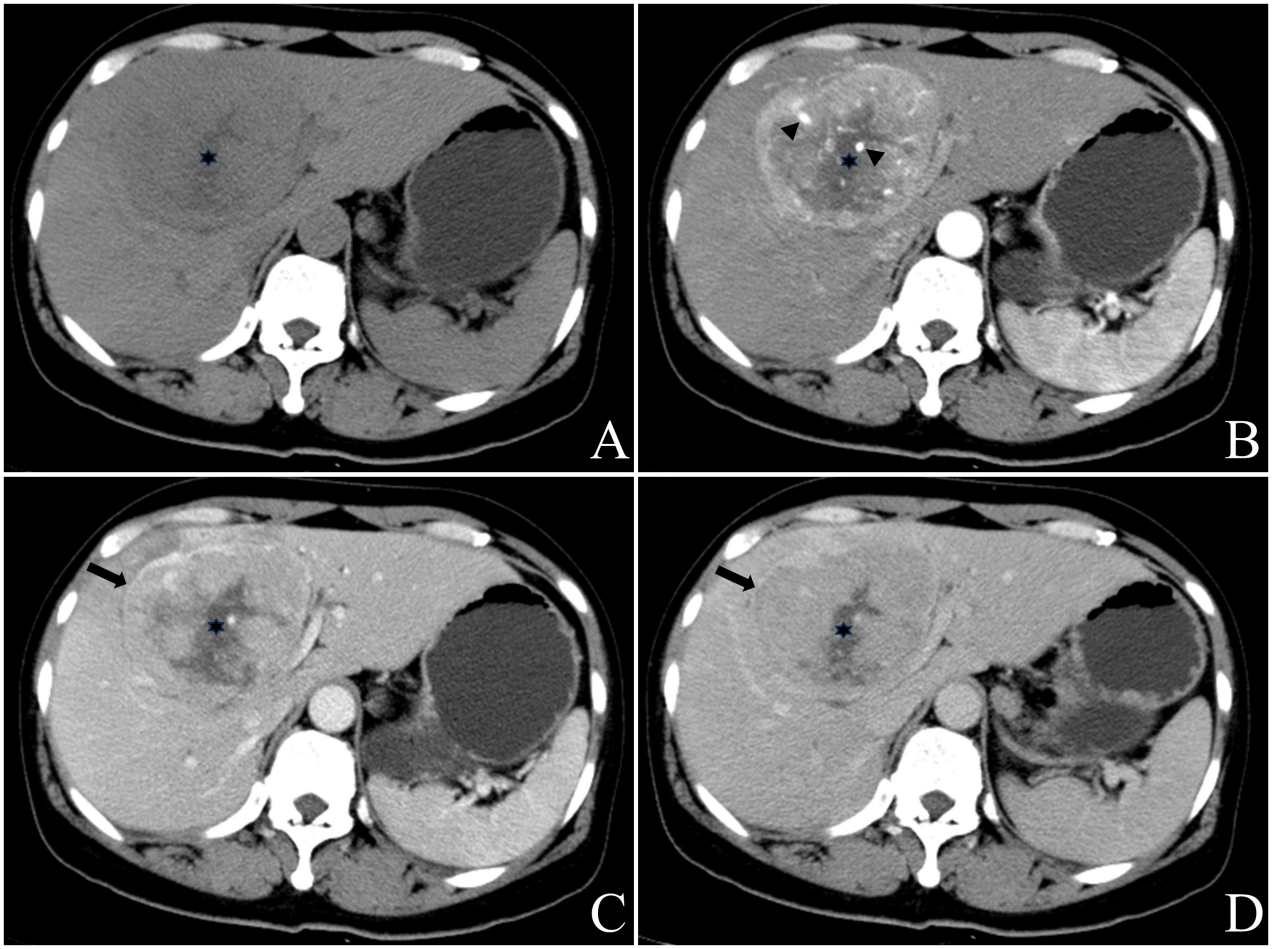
Female, aged 50 years, presented with right upper quadrant abdominal tightness and pain persisting for five days along with abdominal distention, occasional dizziness, and fatigue. Liver-related tumor markers as well as NSE levels were within normal range. A preliminary diagnosis of hepatocellular carcinoma with multiple metastases was made based on contrast-enhanced CT findings in May 2018. The masses located at hepatic S4 and 8 displayed a round mildly lobulated mass measuring up to a maximum cross-section size of approximately 90.4mm x80.5mm; it had well-defined boundaries and appeared slightly heterogeneous hypodense on pre-contrast CT images. Irregular circumferential hyperenhancement was observed on arterial phase imaging along with thickened and thickened tumor blood vessels (B), black arrow head), while peripheral “washout” on venous portal phase and delayed phase accompanied by incomplete capsule (C, D), black arrow). A radially low attenuation area with no apparent enhancement is seen in the center of the mass (A-D), black asterisk). CT value of the most obvious enhanced area/normal hepatic parenchyma: pre-contrast 46.3/52.1; arterial phase 95.0/62.2; venous portal phase 92.9/101.8; delay phase 78.4/87.6. Enlarged right half liver resection was performed in May 2018. Postoperative pathology: neuroendocrine carcinoma (G3). EP chemotherapy regimen was performed after surgery, and the patient remains alive with no evidence of extrahepatic NENs.
